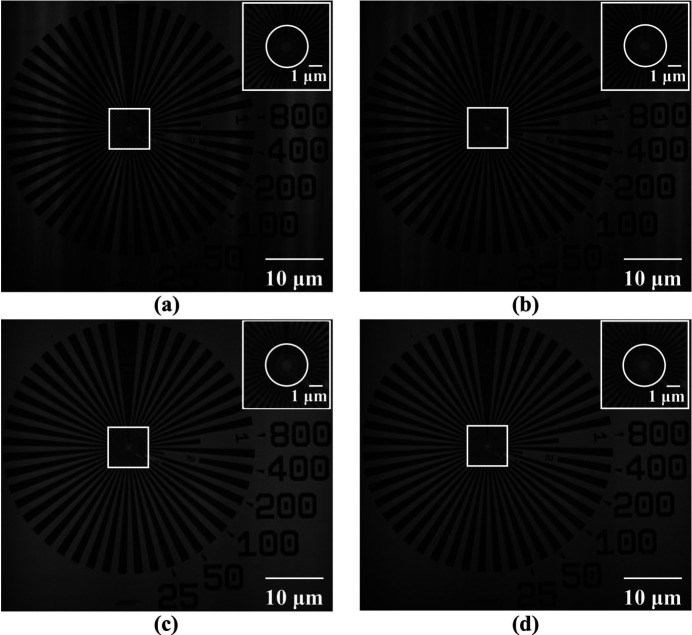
X-ray imaging results with defocus distances of 0 and 1 mm for two secondary light-source sizes: (*a*)–(*b*) 400 µm × 20 µm and (*c*)–(*d*) 400 µm × 80 µm using the beam-shaping condenser.
